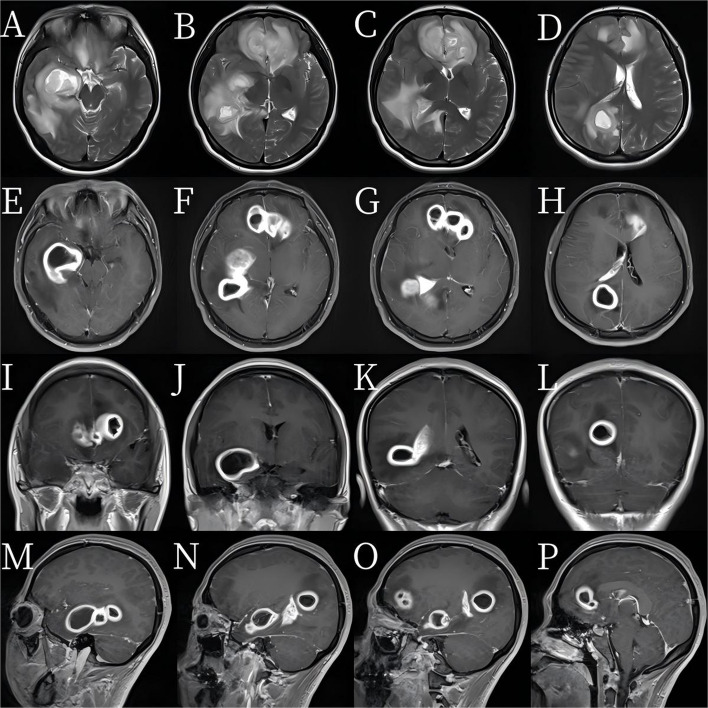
A–D Multiple mass-like hyperintense lesions on T2-weighted images are seen in the bilateral frontal lobes, right temporal lobe, and right occipital lobe. E–P Contrast-enhanced MR image showing prominent ring enhancement of the lesions, surrounded by extensive T2 hyperintense edema
